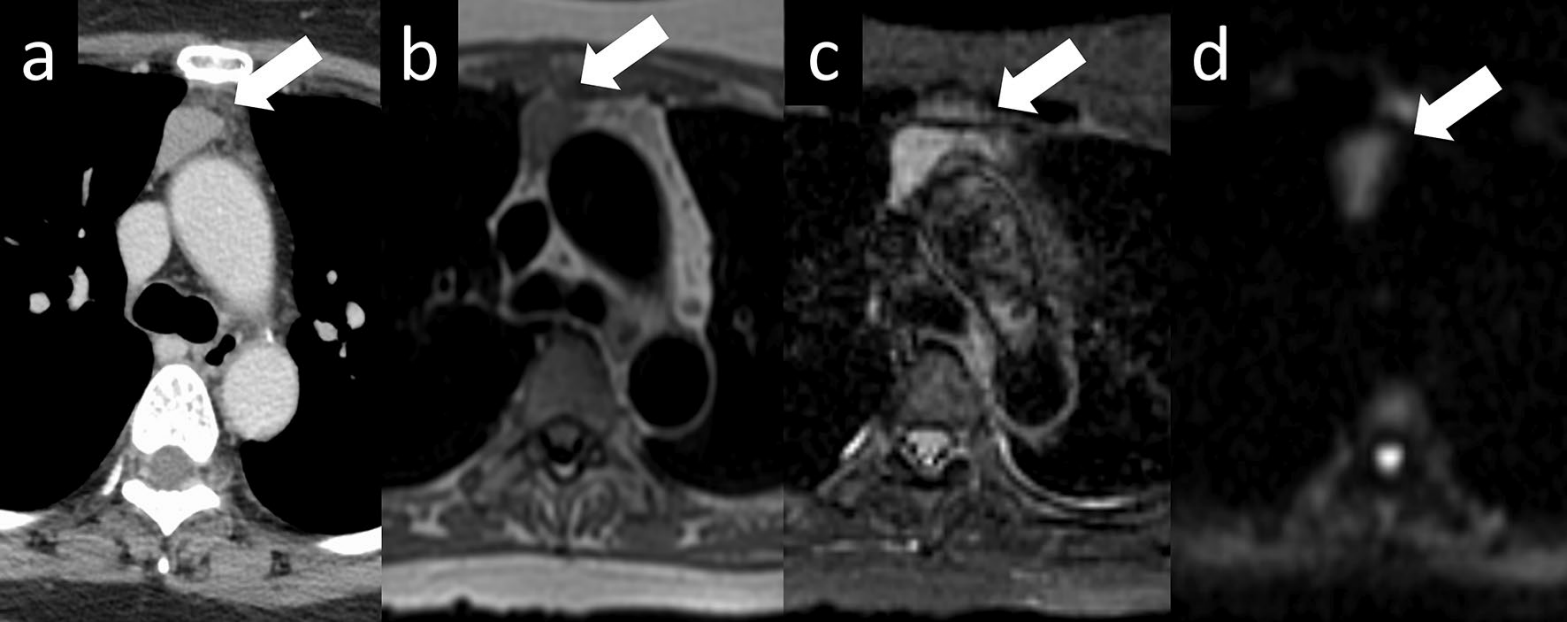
Thymoma (type AB). A 50-year-old woman with type AB thymoma. a Chest CT scan shows a 2-cm prevascular nodule with well-defined borders (arrow). b–d MRI shows a slightly indistinct border with perivascular fat on the left side of the nodule and a slightly low ADC value (1.36 × 10^–3^ mm^2^/s) (arrow) (b: T1WI, c: fat-suppressed T2WI, d: DWI). CT, computed tomography; MRI, magnetic resonance imaging; ADC, apparent diffusion coefficient, T1WI, T1-weighted image; T2WI, T2-weighted image; DWI, diffusion-weighted imaging
